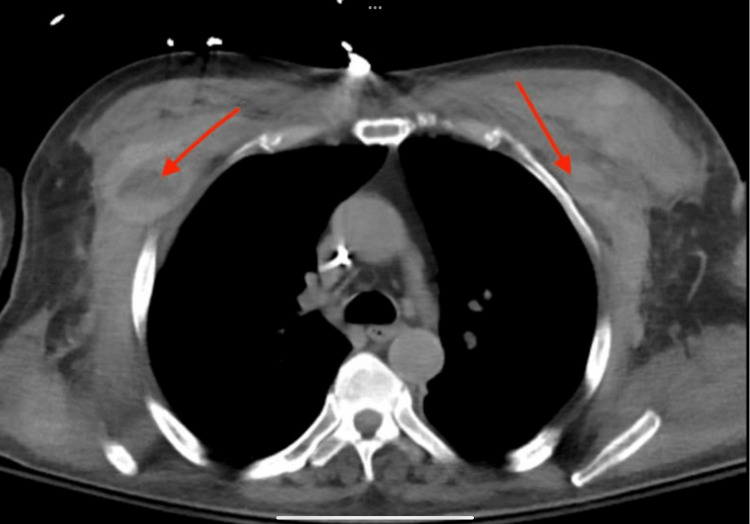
A non-contrast CT scan of the chest revealing bilateral thickening of the pectoralis muscles, with more pronounced involvement on the right side and a fluid collection measuring 2.5 cm × 1.3 cm.
